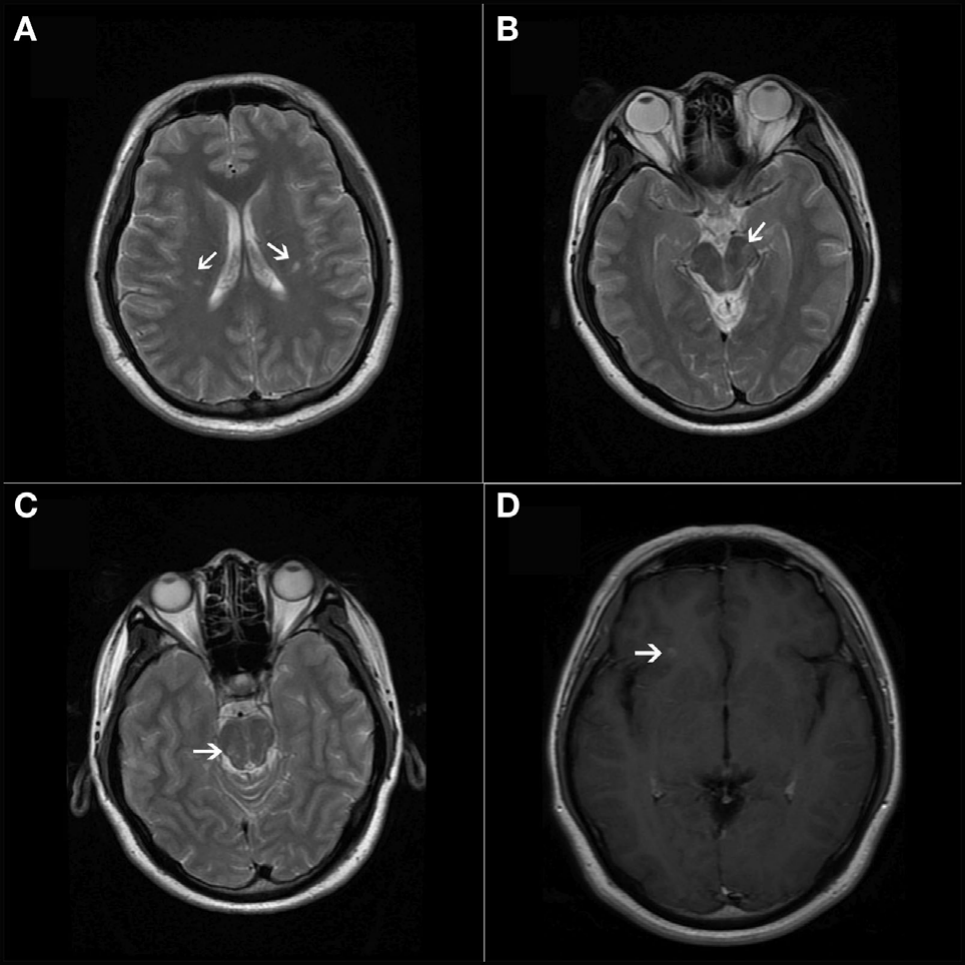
(A–C) T2-weighted axial magnetic resonance imaging showed lesions in bilateral periventricular and infratentorial areas of the CNS. (D) T1-weighted contrast-enhanced image showed a simultaneous presence of asymptomatic gadolinium-enhancing lesion at the juxtacortical area of the anterior part of the insular cortex.
